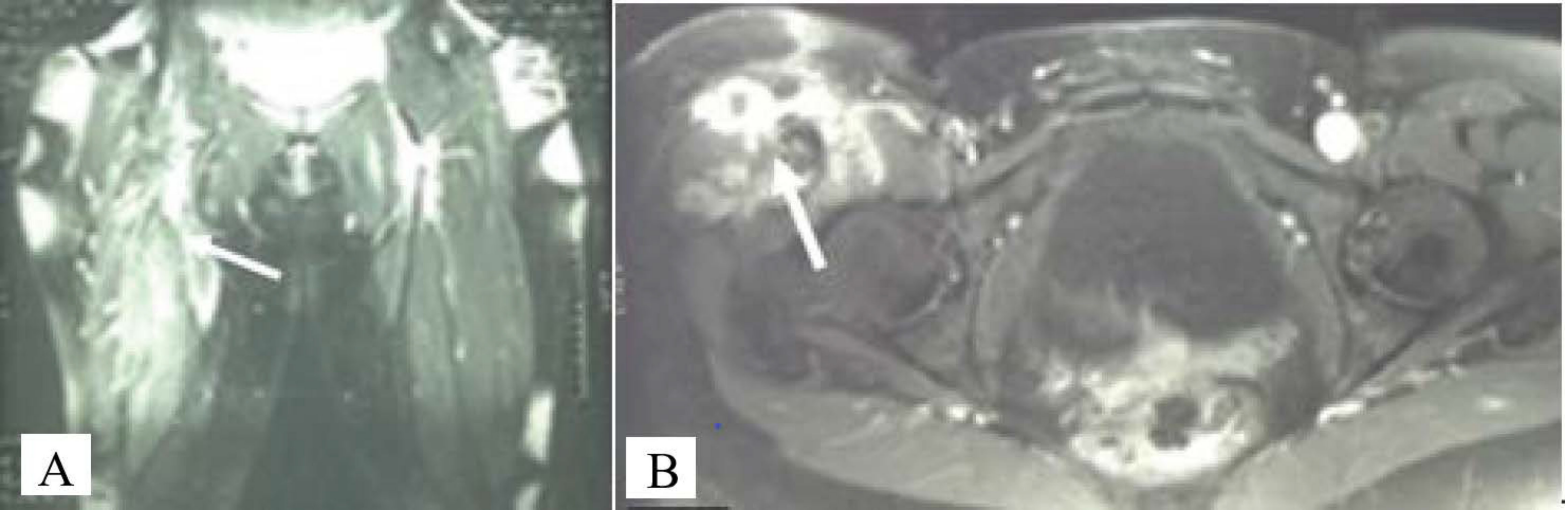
(A) Coronal T2-weighted magnetic resonance image of bilateral femurs taken in June 2004, with arrow showing ill-defined mass with intramuscular signal abnormalities involving the right proximal rectus femoris, vastus intermedius, and vastus lateralis muscles. (B) Axial T2-weighted magnetic resonance images of bilateral femurs taken in June 2005 with arrow showing progression of disease and new involvement of the sartorius, quadriceps, vastus lateralis, and iliopsoas with the tumor also now circumferentially encasing the femoral nerve.
